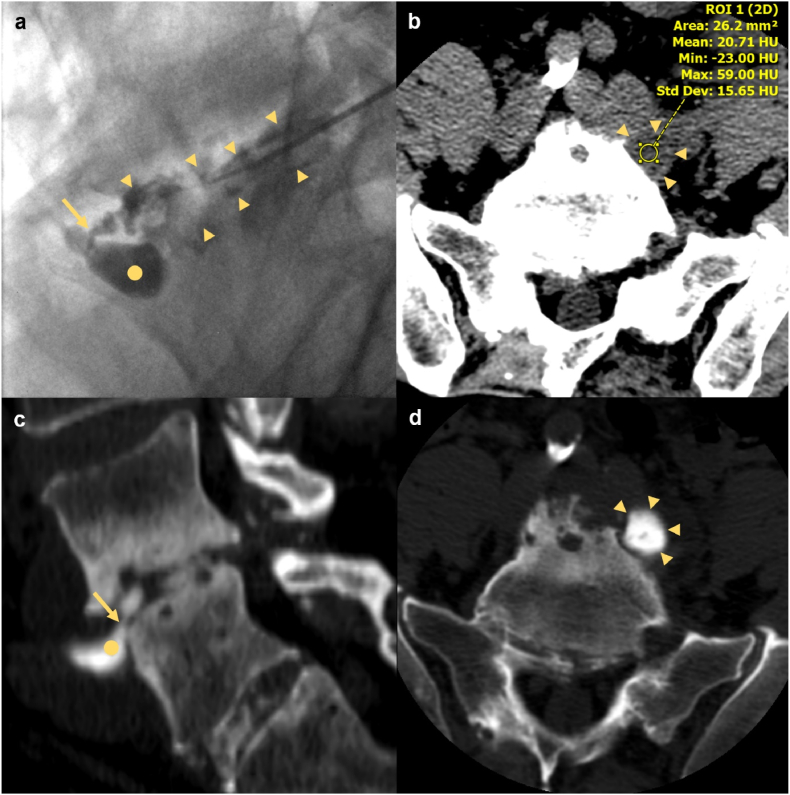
CT and fluoroscopic-guided discography in a 63 year old male with prior L4-L5 discectomy who presented with 2 years of back and bilateral leg pain. (a) Lateral fluoroscopic projection with a percutaneous needle positioned in the L4-5 disc demonstrates opacification of a ventral paraspinous discal cyst (dot) which clearly communicates with the L4-5 disc space (arrowheads) through a narrow neck (arrow). (b) Axial unenhanced pre-procedural CT reveals a hypoattenuating (21 HU) cystic lesion in the left anterior paravertebral space (arrowheads). (c) Parasagittal post-discography CT shows opacification of the discal cyst (dot) which clearly communicates (arrow) with the opacified L4-5 intervertebral disc. (d) Axial post-discography CT shows opacification of the cystic lesion with contrast (arowheads). Approximately 3 mL of serosanguinous fluid was aspirated from the cyst.
